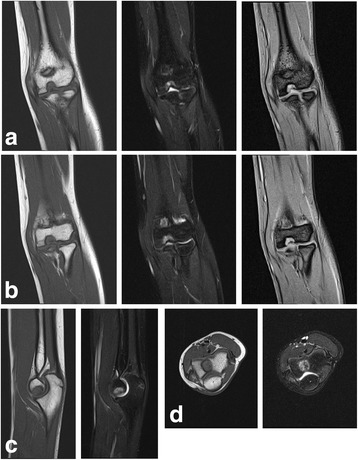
A 14-year-old girl with distal defect of the humerus. Coronal magnetic resonance imaging shows low-signal to iso-signal intensity on T1-weighted images (*left*) and slightly high-signal intensity on T2-weighted fat suppression (*middle*) and short tau inversion recovery images (*right*) (a and b, sequential sections). Sagittal (c) and coronal (d) sections are present (*left*, T1-weighted image; *right*, T2-weighted image)
